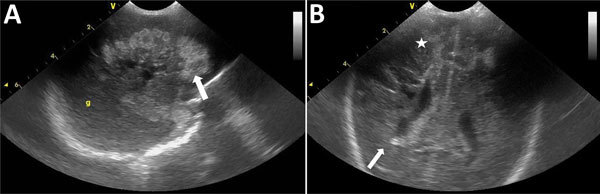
Standard echography cranial ultrasound of premature infant with *Bacillus cereus* sepsis, Nice, France, 2013. A) Left sagittal section showing large hemorrhagic hyperechogenic area of white material (white arrow). B) Frontal section showing right periventricular kystic hypoechogenic lesions (white arrow) with associated bilateral hemorrhagic hyperechogenic lesions (white star).
